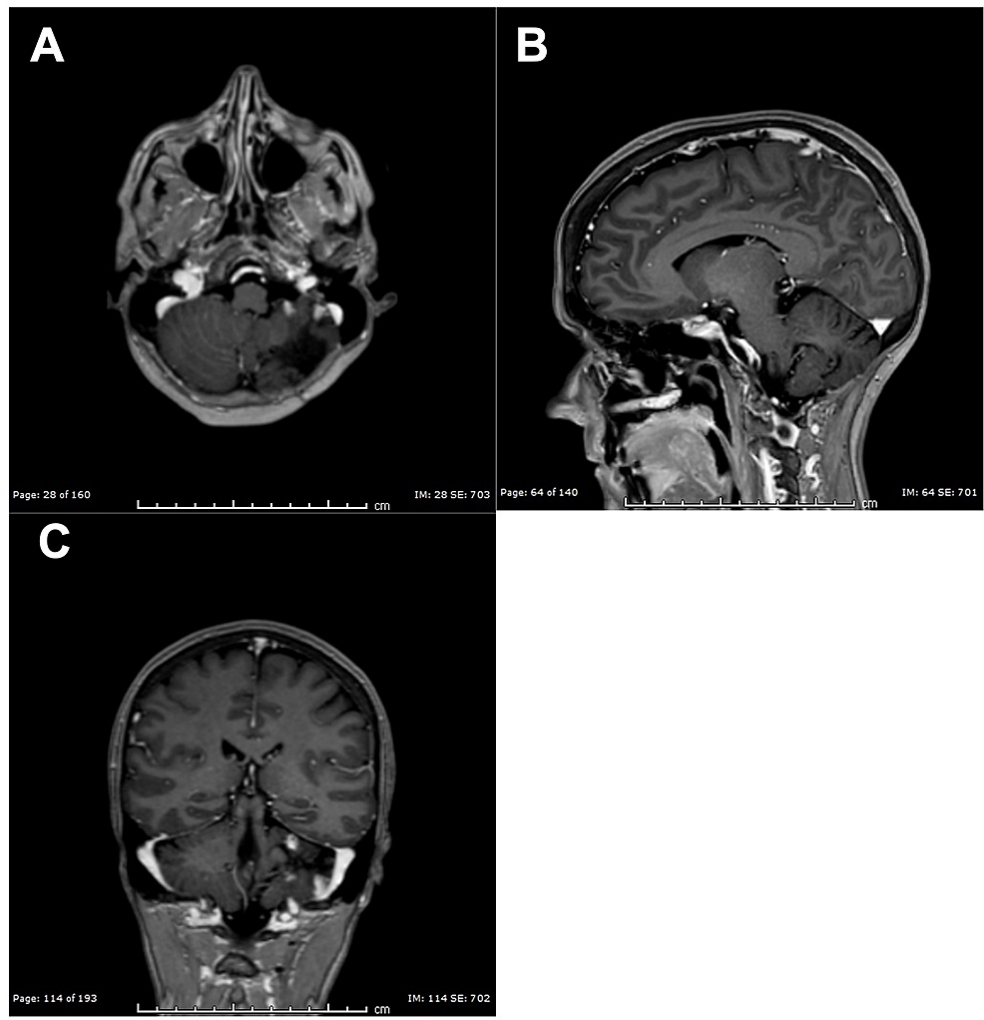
MR Images of Resolution of Leptomeningeal Carcinomatosis (A) Axial T2 MR image demonstrating resolution of leptomeningeal carcinomatosis with stable cerebellar lesions. (B) Sagittal T1 MR image with gadolinium contrast demonstrating resolution of leptomeningeal carcinomatosis with stable cerebellar lesions. (C) Coronal T1 MR image with gadolinium contrast demonstrating resolution of leptomeningeal carcinomatosis with stable cerebellar lesions.
